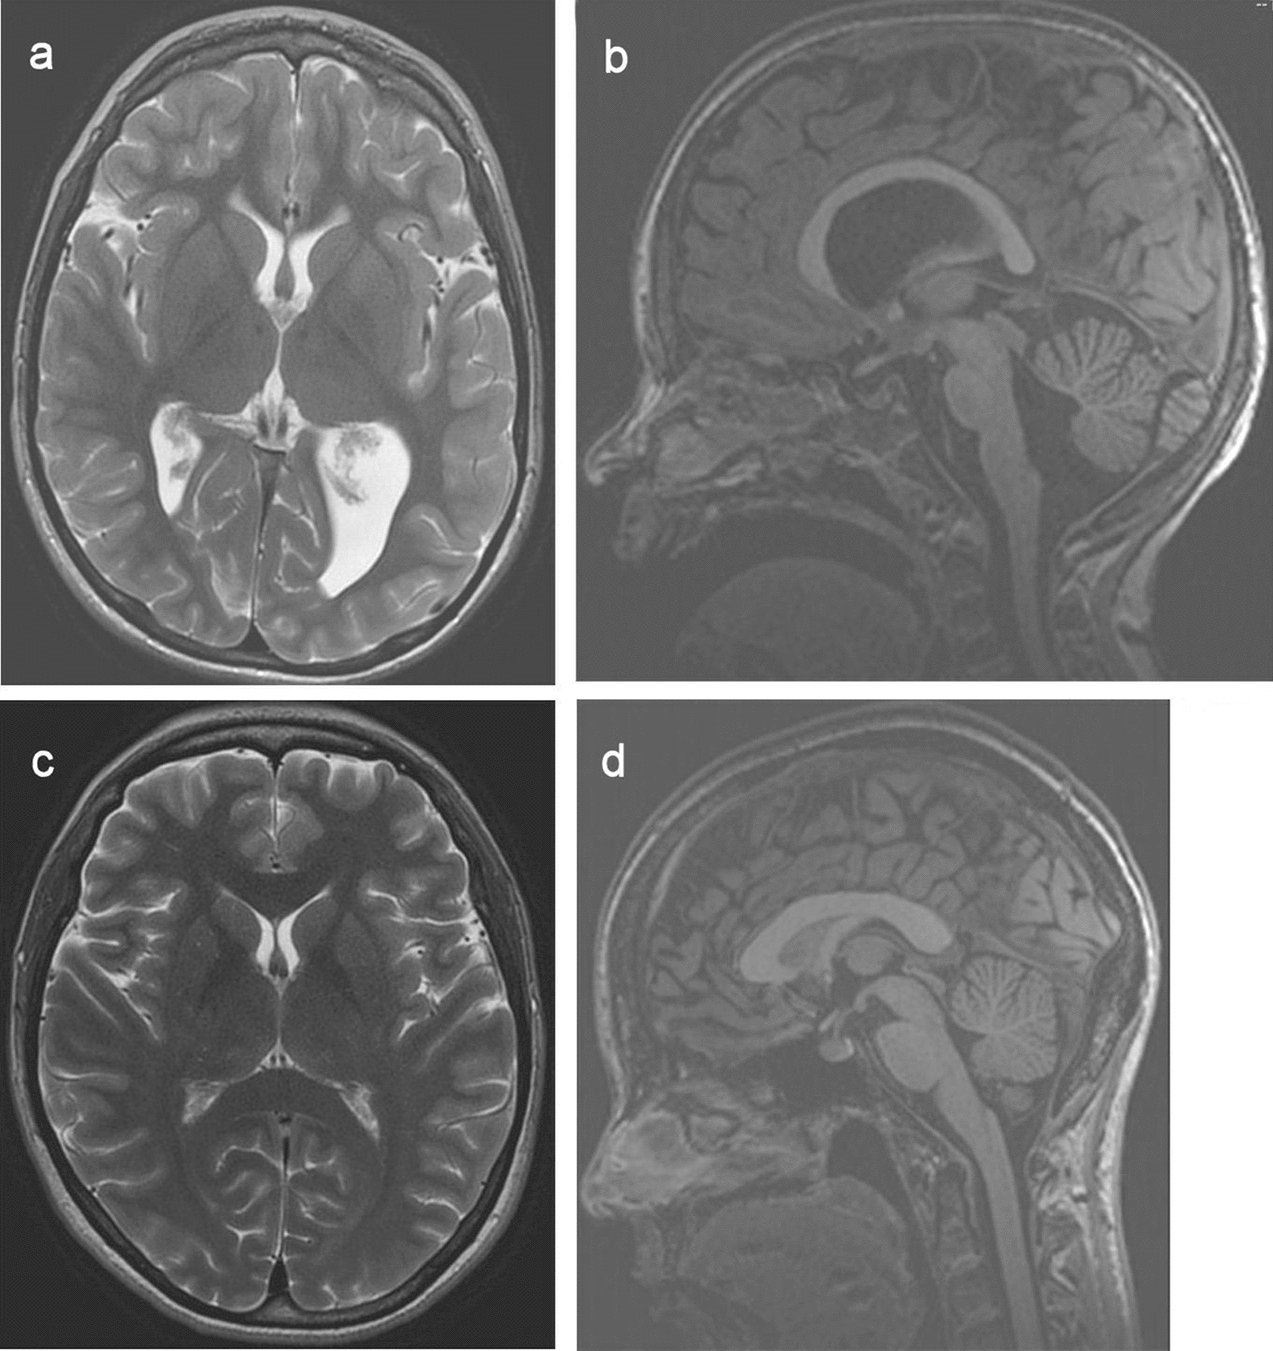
a MRI in the axial plane, T2–WI (proband). b MRI in the sagittal plane, T1–WI (proband). There is moderate thinning of the corpus callosum, reduced size of the pons, and aplasia of lower segments of the cerebellar vermis (Dandy–Walker variant); chiasm, quadrigeminal, interpeduncular, more closely tank expanded. c MRI in the axial plane, T2-WI (mother); no pathology detected. d MRI in the sagittal plane, T1–WI (mother). No pathology detected
